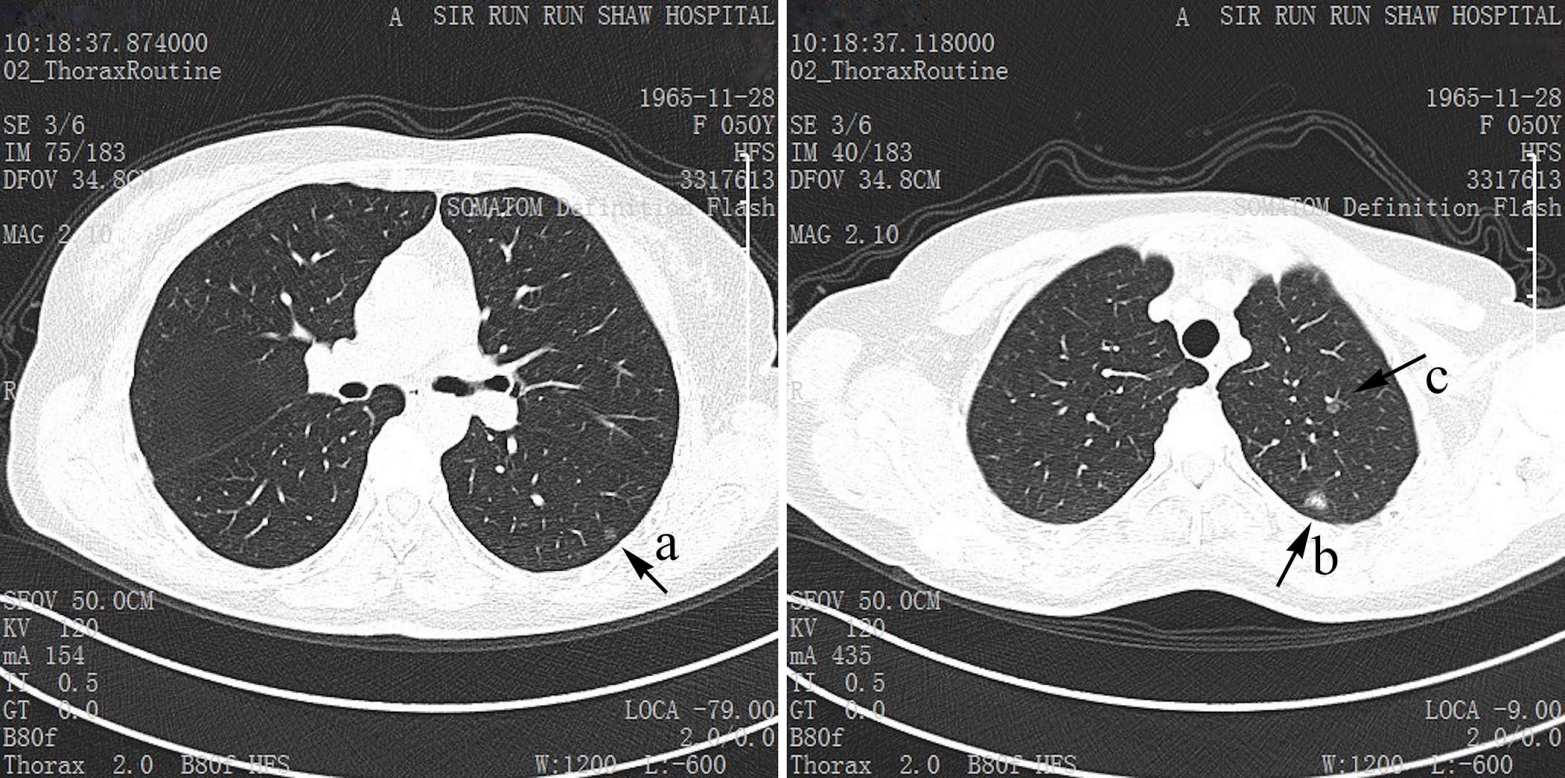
The typical CT images of AIS and MIA The nodule a and c are typical AIS, and the larger nodule b in the left upper lobe is MIA.
